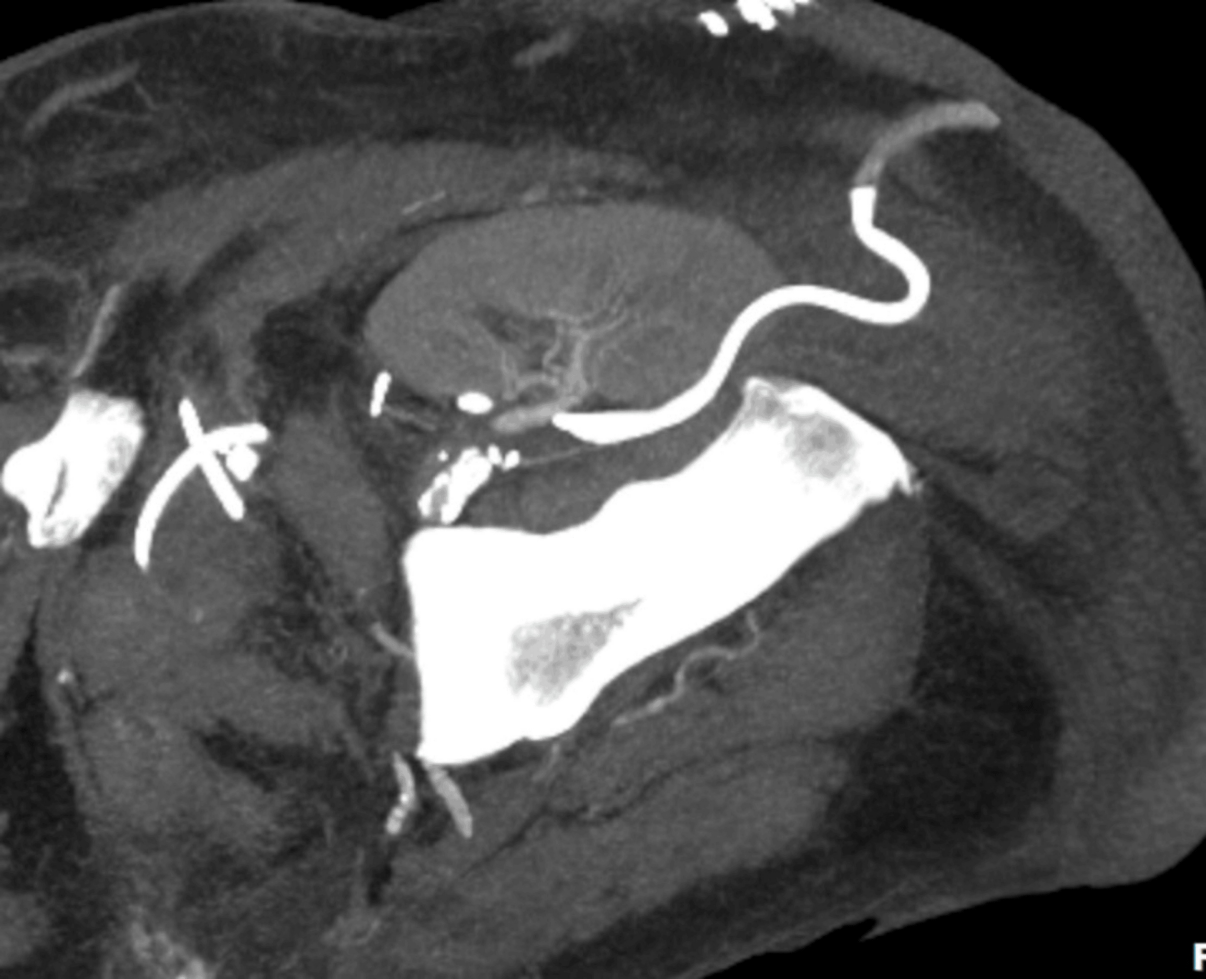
CT angiography demonstrating adequate perfusion of the renal graft. CT, computed tomography
